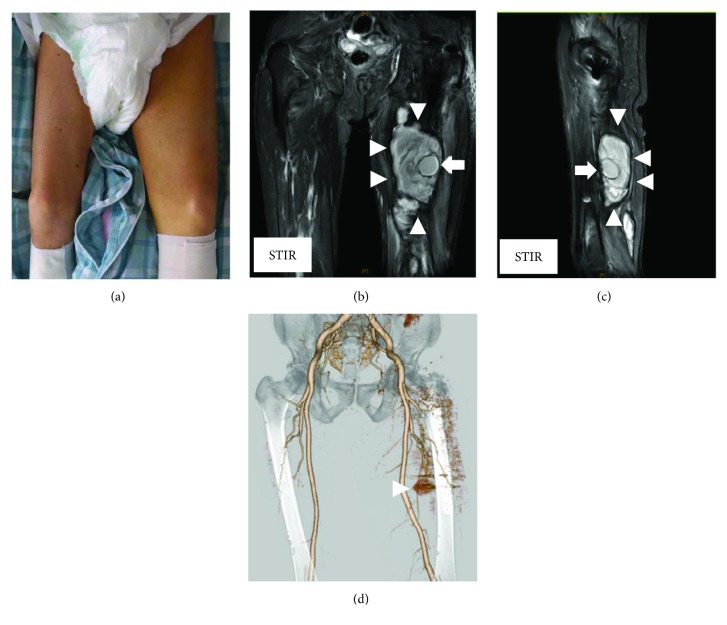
(a) Swelling of the affected limbs. (b, c) Magnetic resonance imaging shows pseudoaneurysms (arrow) and huge hematoma (arrowhead). (d) Computed tomography angiographies with a 3-dimensional reconstruction view demonstrate pseudoaneurysms (arrowhead).
